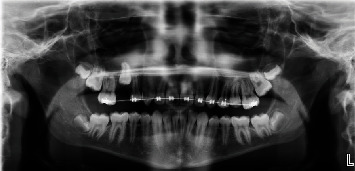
Progress panoramic radiograph, twelve months after extraction of #A.
